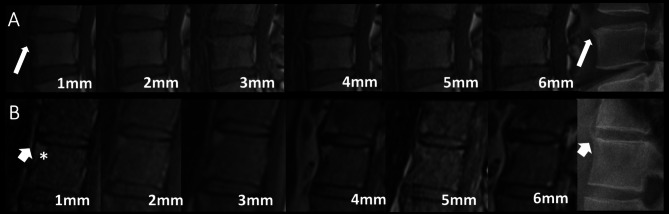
(A) Syndesmophyte at the anterior superior corner of L4 detected on both each MRI slice thickness and radiography (thin arrow). No concurrent presence of fat at the same corner. (B) Syndesmophyte at the anterior superior corner of Th12 detected on both each MRI slice thickness and radiography (thick arrow). Concurrent presence of fat at the same corner in MRI (asterisk) L: Lumbar; MRI: Magnetic Resonance Imaging; Th: Thoracic
